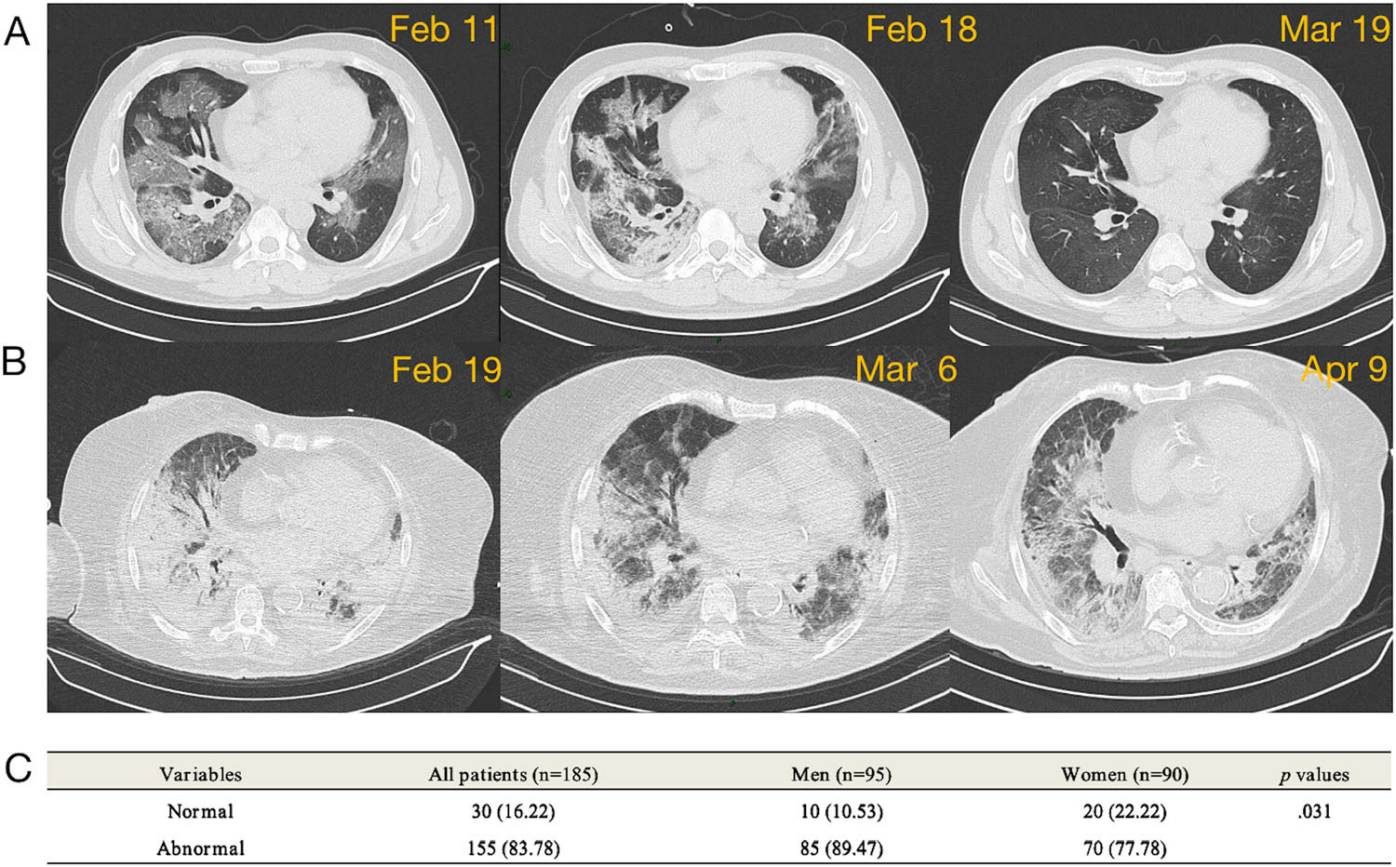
Chest computed tomographic (CT) images. (A) Chest CT images of a male patient infected with SARS‐CoV‐2 were partially absorbed after nasal high‐flow oxygen inhalation treatment. (B) Chest CT images of a female patient infected with SARS‐CoV‐2 showed patchy ground glass shadows and diffuse pulmonary fibrosis. (C) Higher percentages of CT abnormalities were discovered in male patients at the first examination after admission
